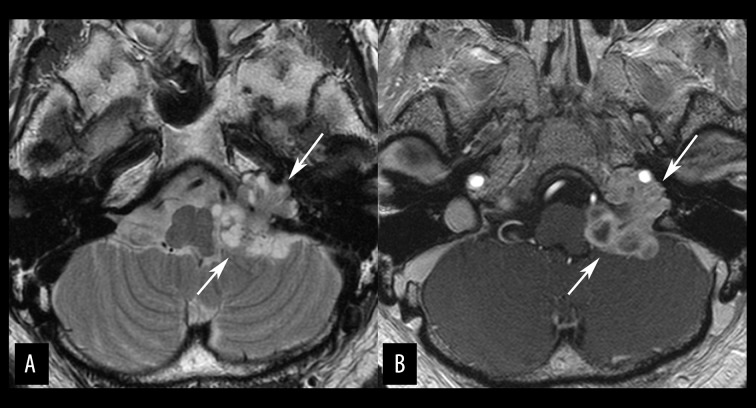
Jugular foramen schwannoma in a 66-year-old man. (A) Axial, T2-weighted image shows a mixed solid and cystic mass (arrows) extending through the left jugular foramen. (B) Axial, post contrast, T1-weighted image shows heterogeneous enhancement of the tumor (arrows).
